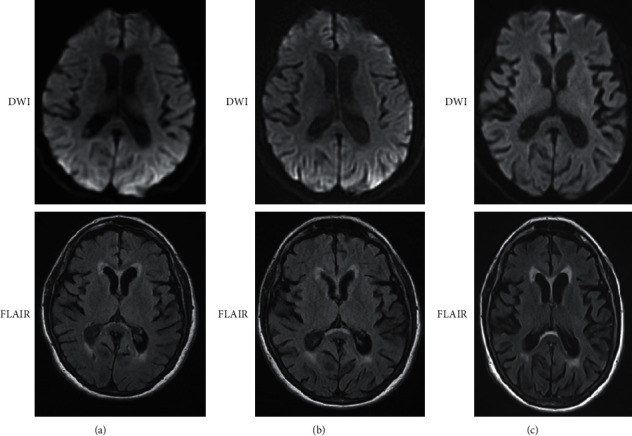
Axial MRI diffusion weighted images (top row) and fluid-attenuated inversion recovery images shown at about one month (a), four months (b), and nine months (c) from onset of symptoms. These images show no diffusion restriction on diffusion weighted images or hyperintensities on FLAIR that would support the diagnosis of Creutzfeldt–Jakob disease.
